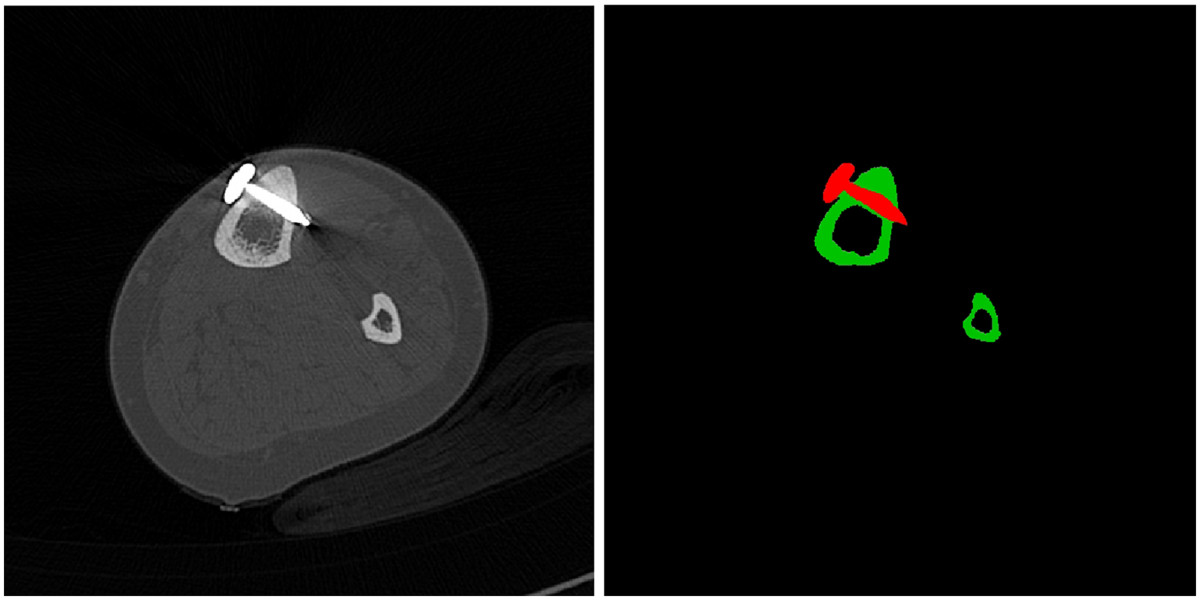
Original computed tomography image (left); results of the segmentation process (right).
